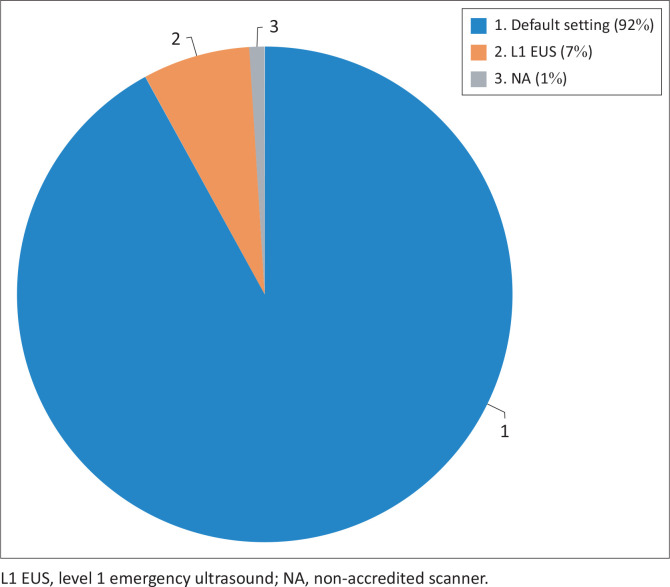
Point-of-care ultrasound scanning by level of point-of-care ultrasound accreditation.
